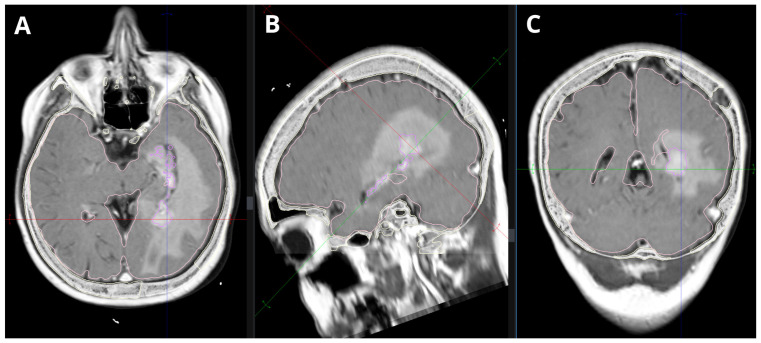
MPR merge CT + post contrast T1-weighted MRI showing an intraventricular homogeneously contrasted tumor inside the temporal horn of the left lateral ventricle. The tumor is growing along the choroid plexus. (A) Axial plane. (B) Saggital plane. (C) Coronal plane.
